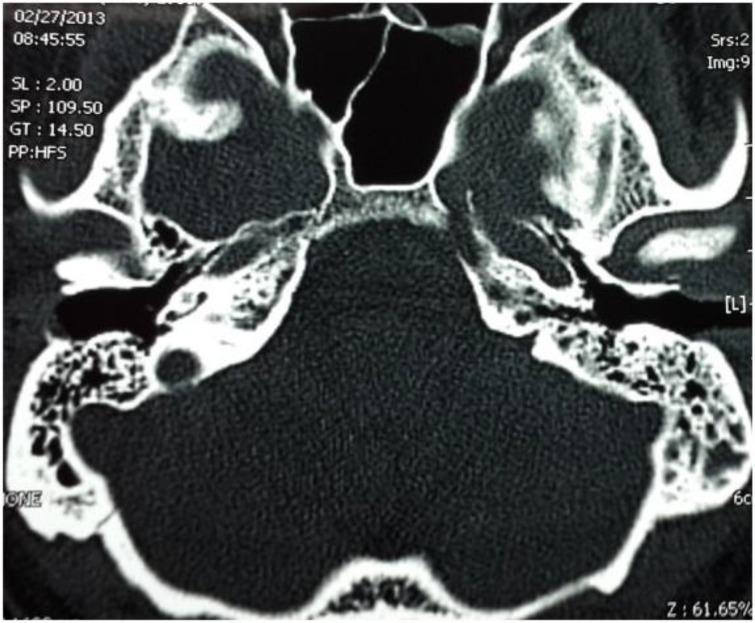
Axial temporal bone CT image showing disappearance of opacification and improvement of aeration at left petrous apex and mastoid bone
